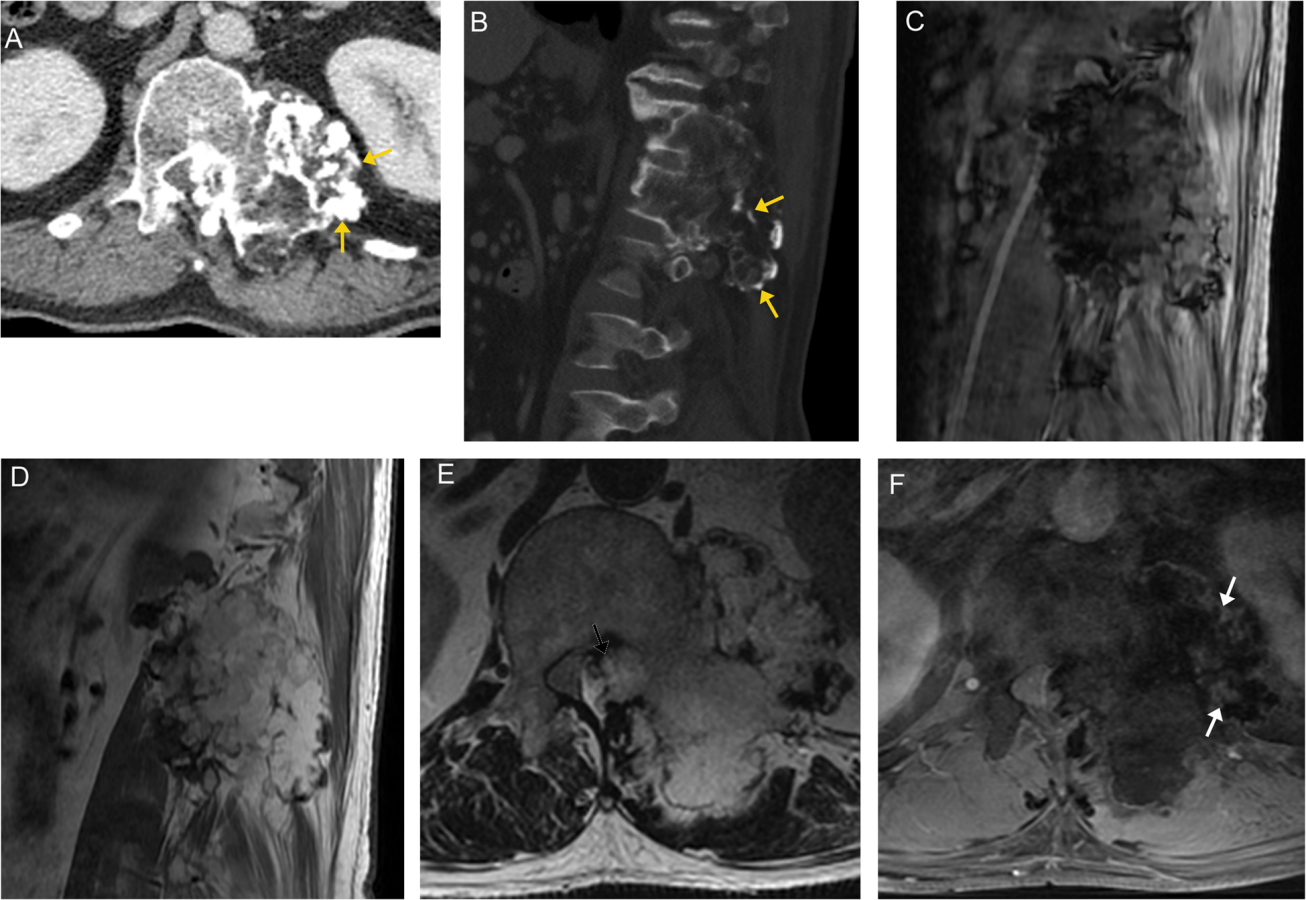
Chondrosarcoma. Axial CT image in the soft tissue window (a) demonstrates a mass centered at the left paravertebral region with expansile ring and arc calcifications (arrows), typical of a chondroid tumor matrix. On sagittal off-midline CT image (b), the tumor is seen extending over multiple vertebral levels. Sagittal GRE image (c) demonstrates areas of low signal within the tumor, due to its calcific components. Sagittal T1-weighted image (d) demonstrates variable signal intensity of the tumor. Axial T2-weighted image (e) demonstrates lobular, predominantly high signal intensity of the tumor due to the high-water content of hyaline cartilage. Additionally, there is epidural extension of the tumor (black arrow), displacing the spinal cord. Peripheral areas of low signal intensity are due to calcifications. The T1 fat-suppressed post-contrast image (f) demonstrates enhancement along the periphery and internal septa (white arrows). The non-enhancing areas represent hyaline cartilage, cystic mucoid tissue and necrosis
